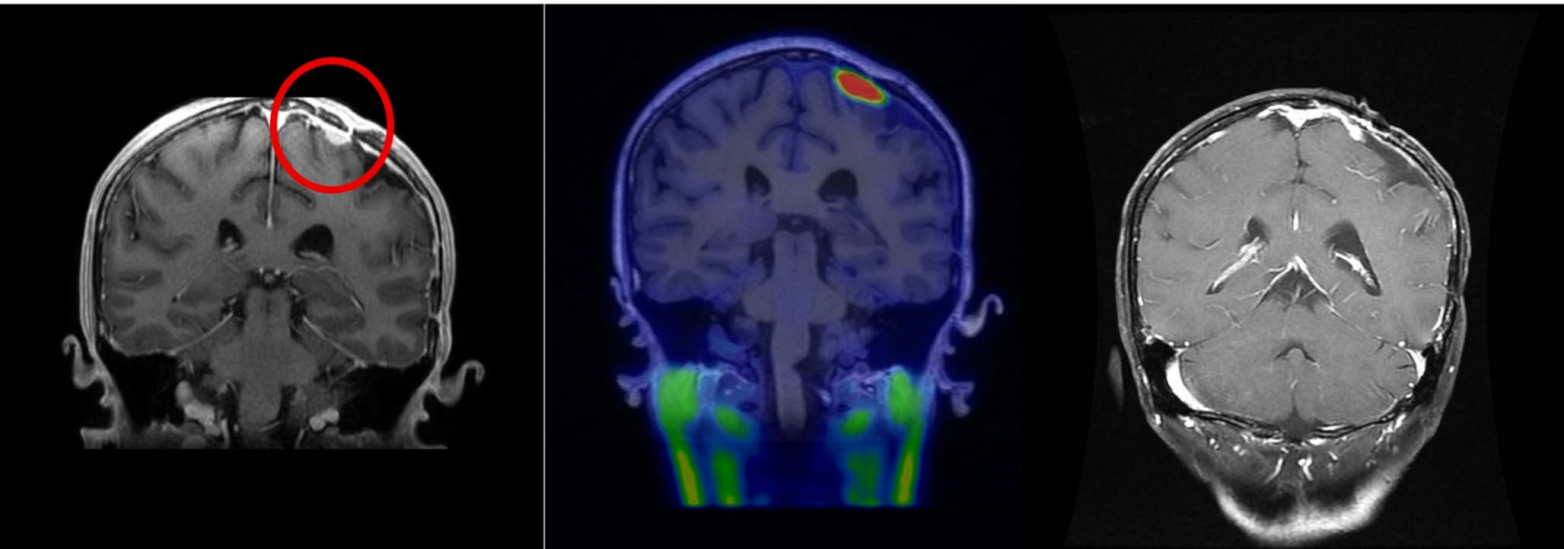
Representative case of a patient with recurrent convexity atypical meningioma. (Left) Coronal MRI image prior to BNCT, with the tumor delineated by a red circle. (Middle) Pre-treatment Fluciclovine PET scan showing high radiotracer uptake; the tumor-to-blood SUV ratio was 11.58, indicating high expression of amino acid transporters. (Right) Coronal MRI image two months after BNCT showing marked tumor shrinkage, consistent with a complete response
